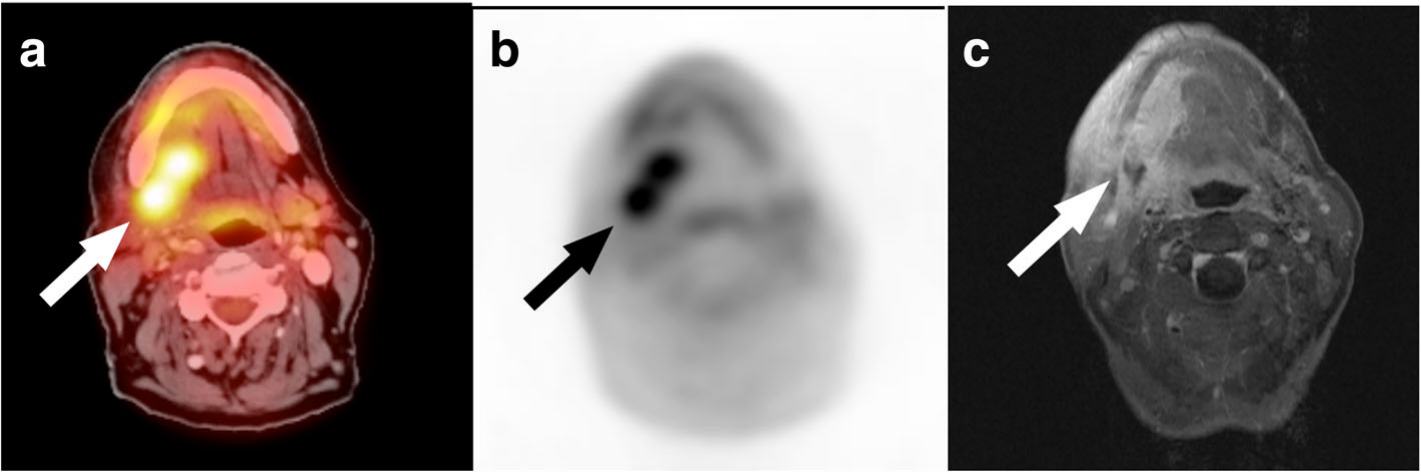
ACC tumour remaining: PET/CT and MR imaging was true-positive. Patient after resection of an adenoid cystic carcinoma, now with a tumour remnant on the right root of the tongue prior to neutron therapy. The tumour mass is seen on the fused PET/CT (a) and PET image (b) with a focally increased FDG-uptake (SUVmax 14.1) and contrast-enhanced T1-weighted MR image (c) (arrow)
